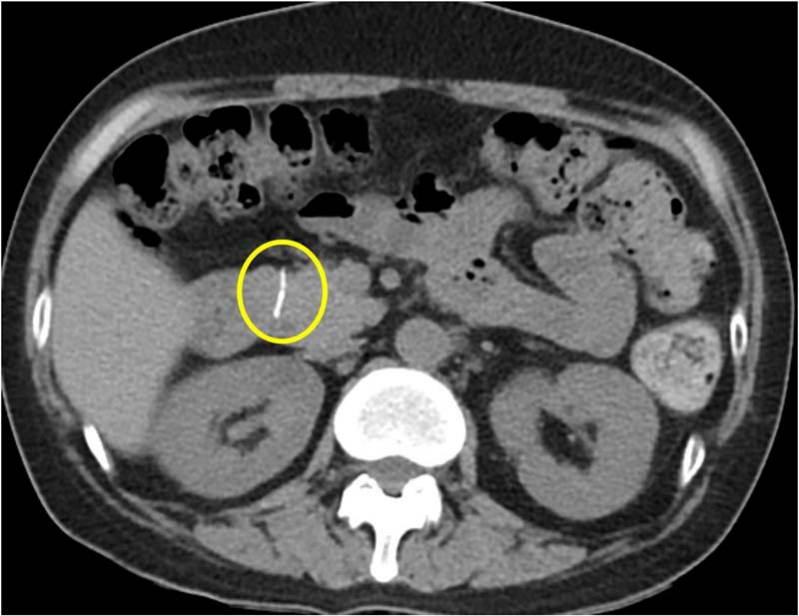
Abdominal CT scan showing a 1.7-cm linear high-density foreign body penetrating the anterior aspect of the head of pancreas.
